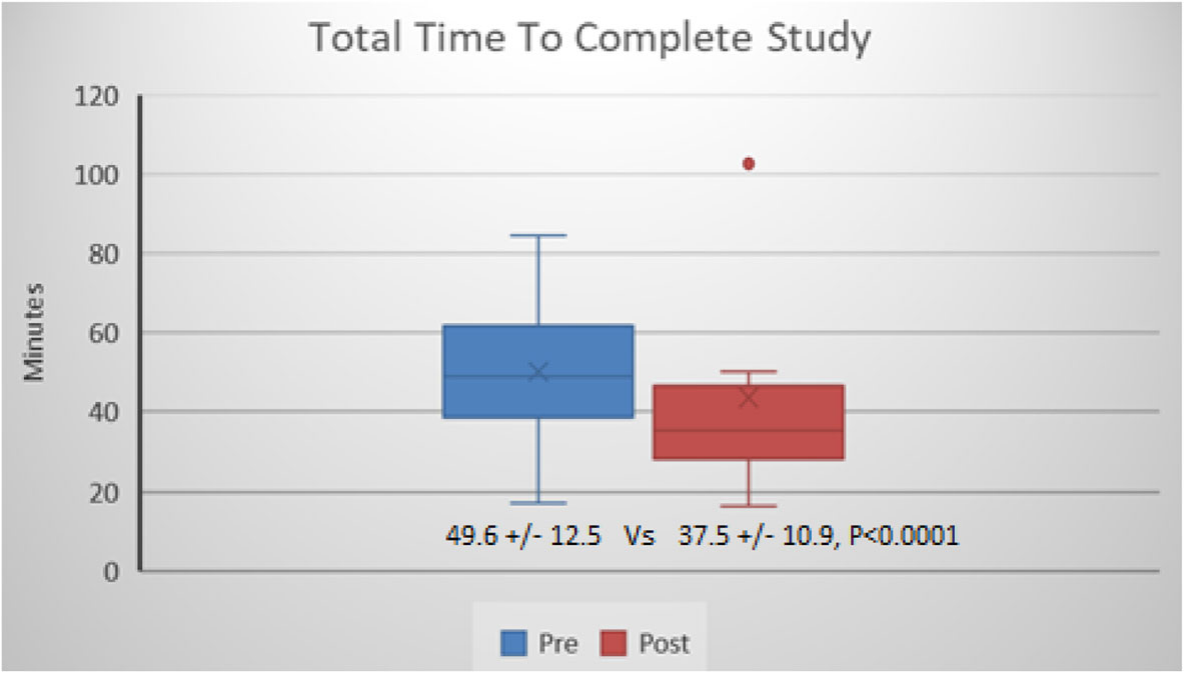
Total Time to Complete Study. Contrast enhanced echocardiograms required 49.6 min using a nurse administered contrast protocol compared to 37.5 min using a sonographer driven protocol, (*P* < 0.0001)
